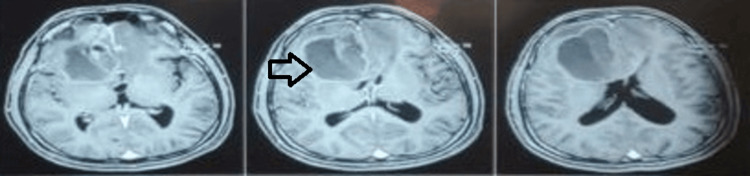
Depicting pre-operative contrast-enhanced T1 MRI images; ring enhancement pattern with extension into the right frontal and ethmoid sinuses.
